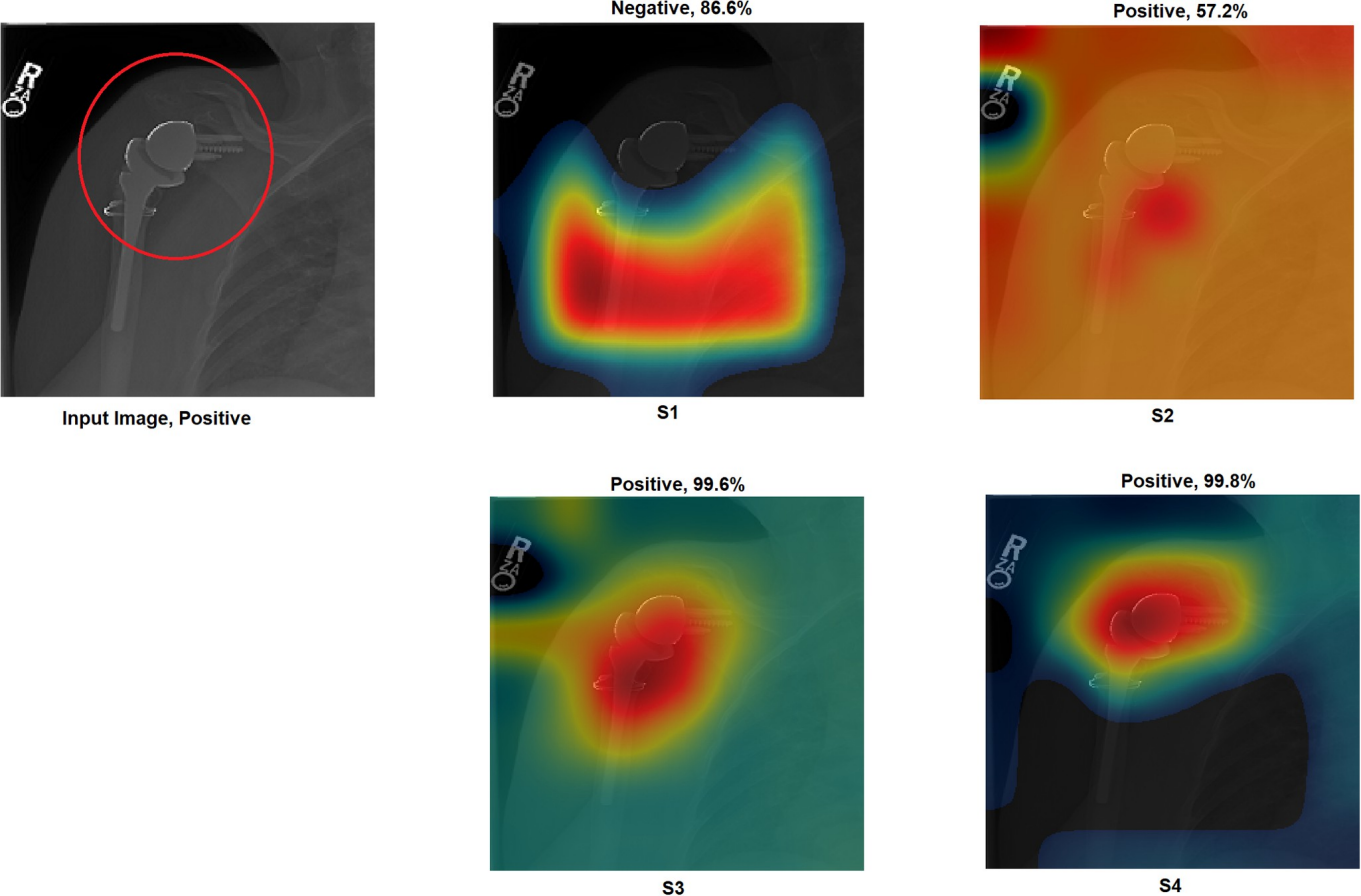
Grad-CAM and Score- Grad-CAM for shoulder X-ray image. The correct classification is Positive. The ROI is the red circle that a domain expert has marked.
